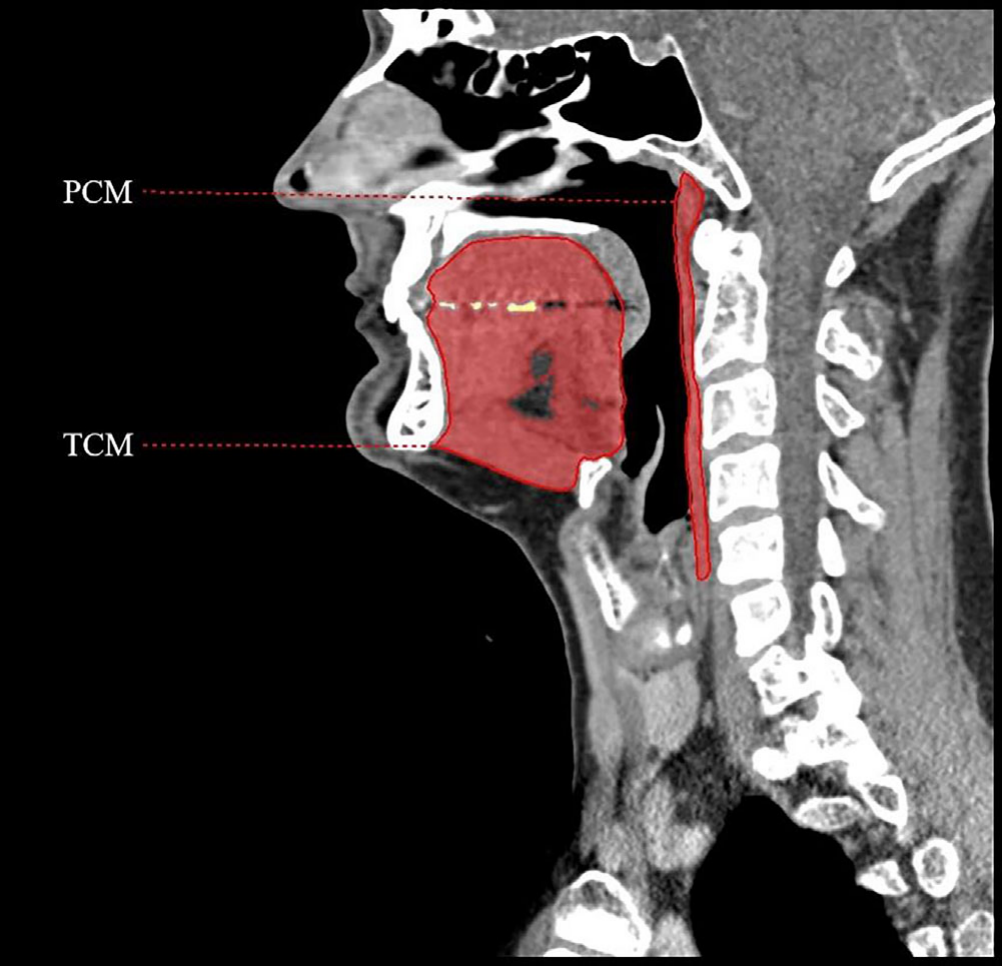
Example of the swallowing muscle mass area measurements on a sagittal computed tomography slice. Anterior, posterior, superior and inferior muscle borders identified according to anatomical landmarks. The red area represents the delineated muscles. PCM, pharyngeal constrictor muscles; TCM, tongue complex muscles.
